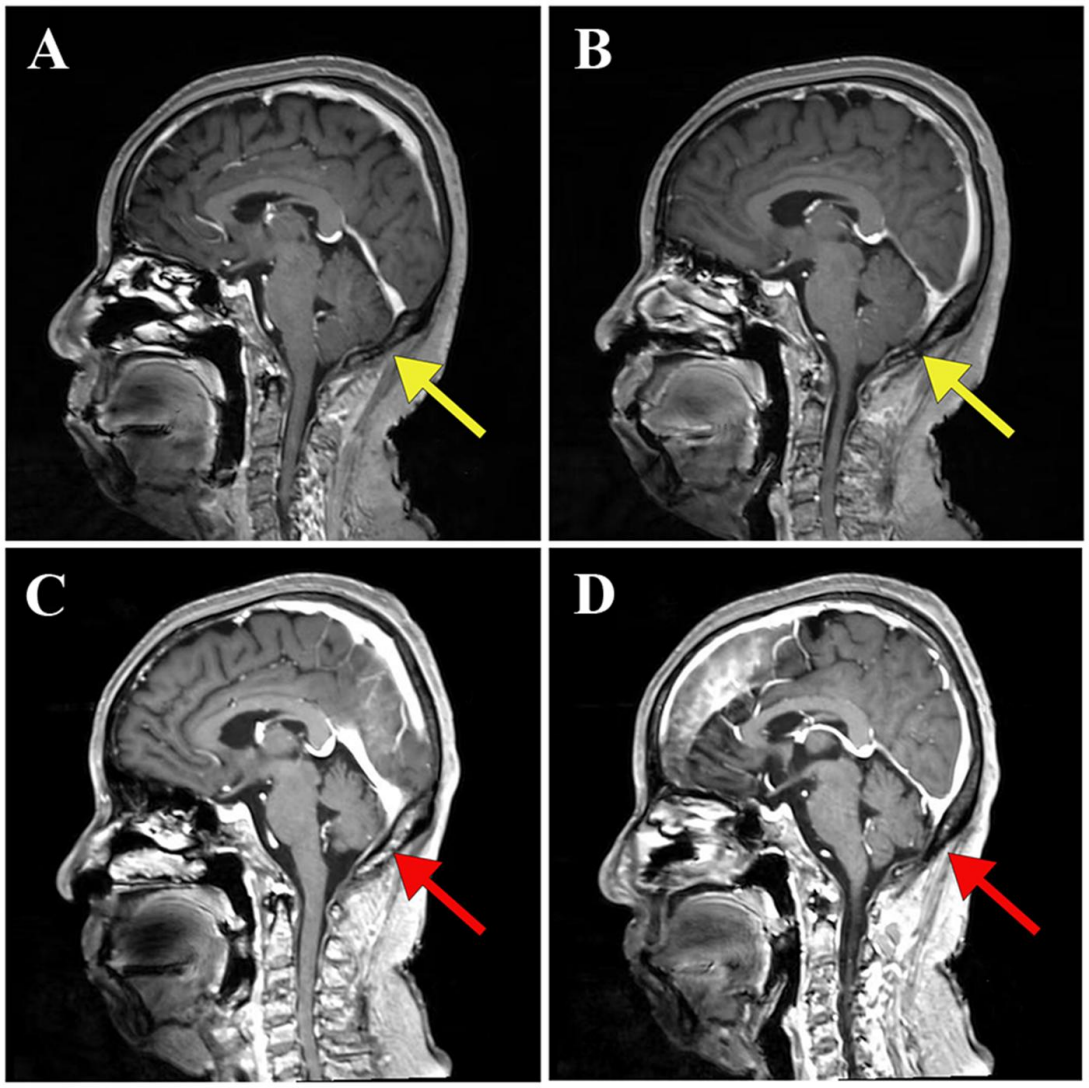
Cranial magnetic resonance imaging of a patient with PCD. (A,B) A cranial MRI conducted on 29 October 2024, revealed no significant abnormalities, as indicated by the yellow arrows. (C,D) A follow-up cranial MRI performed on 25 March 2025, demonstrated notable cerebellar atrophy, as highlighted by the red arrows.
